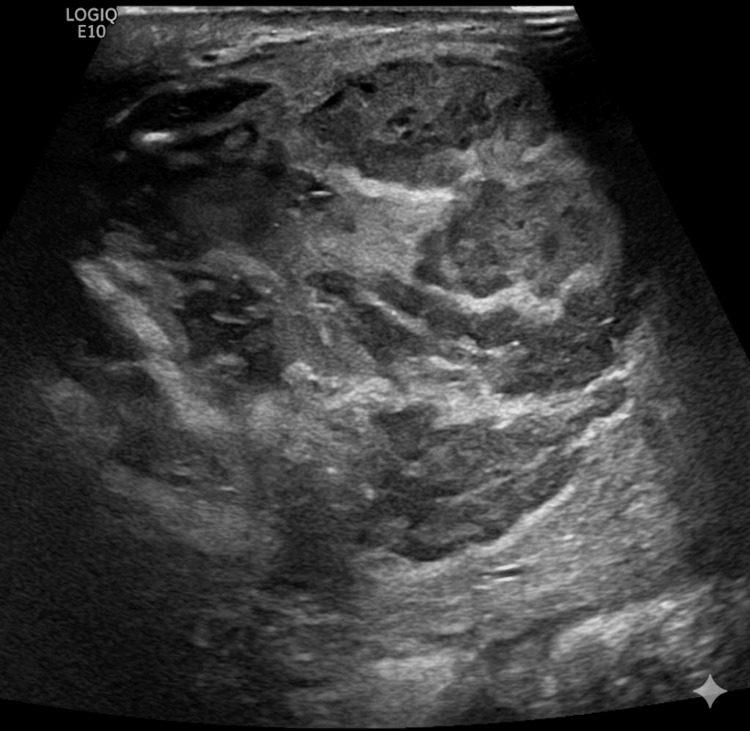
Transverse sonogram of the right scrotum showing a complex, multiloculated fluid collection. The heterogenous appearance and presence of internal echoes are highly suggestive of an abscess. In the context of suspected torsion, these findings likely represent the late-stage inflammatory and necrotic sequelae of prolonged ischemia.
